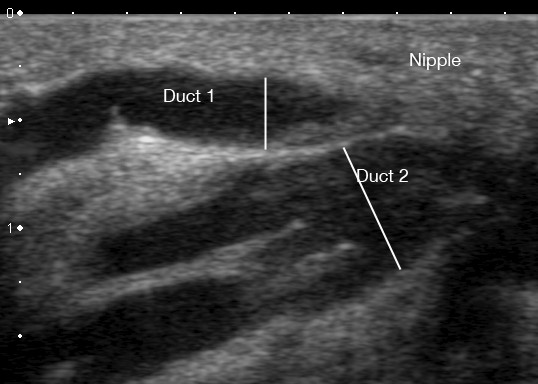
Ultrasound image of a milk ducts in the human lactating breast at milk ejection. Two main milk ducts are displayed on ultrasound as a hypoechoic (black) structure with echogenic walls (white). Duct 1 is more superficial and has increased from 1.95 mm to 3.44 mm in diameter. Duct 2 has increased from 3.72 mm to 6.24 mm.
